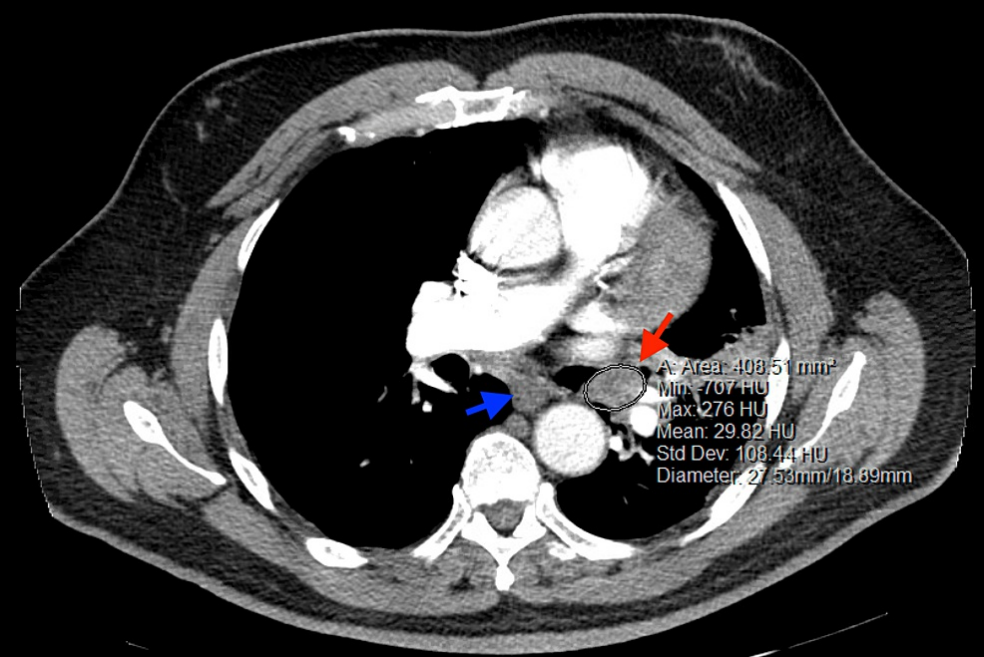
CT chest (axial view) demonstrating the 2.7 x 1.9 cm ovoid mass in the left main-stem bronchus (red arrow) and enlarged mediastinal soft tissue (blue arrow)
